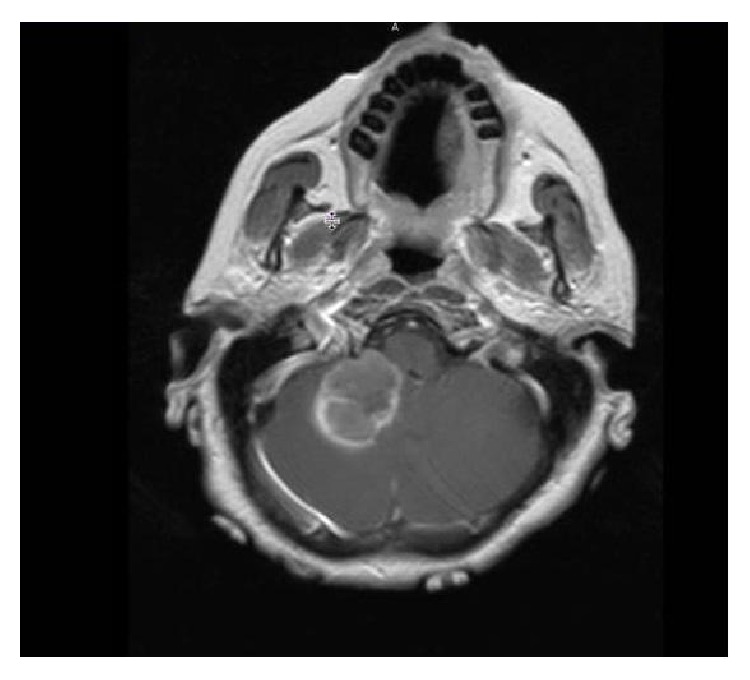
T1 weighted MRI of brain showing right cerebellar lesion.
